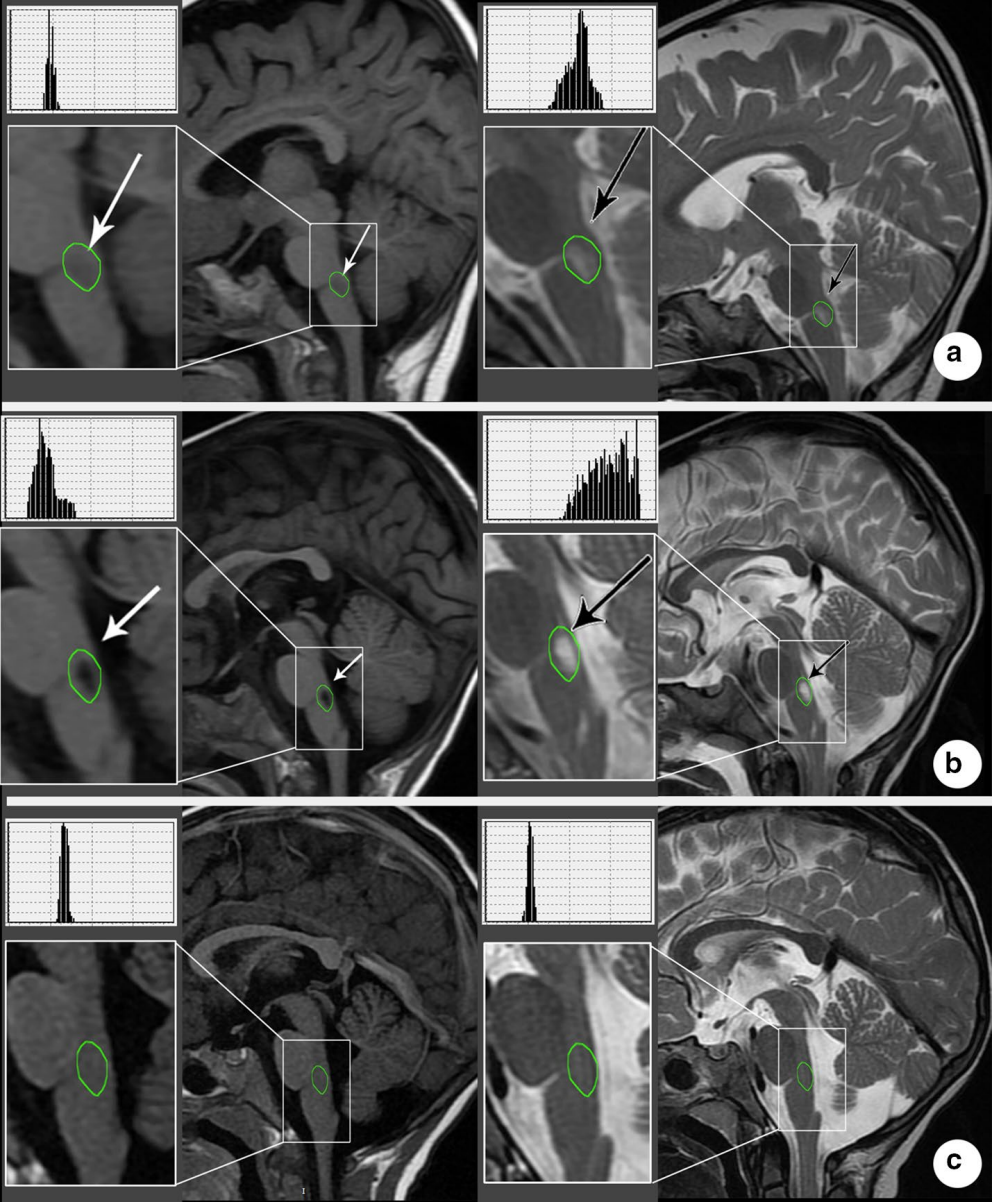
T1WI and T2WI MRI scans of diseased and healthy subjects. a. Acute stage: Sagittal MR images revealed a longitudinal lesion at the posterior junction region of the pons and medulla oblongata, with prolonged T1 (a, *white arrow*) and prolonged T2 (b, *black arrow*) signal. b Convalescence stage: MR images showed there was a longitudinal malacia cavity at the posterior junction region of the pons and medulla, with prolonged T1 (a, *white arrow*) and prolonged T1 (b, *black arrow*) signal. c) Healthy controls: plain MRI scan show there is no abnormality
